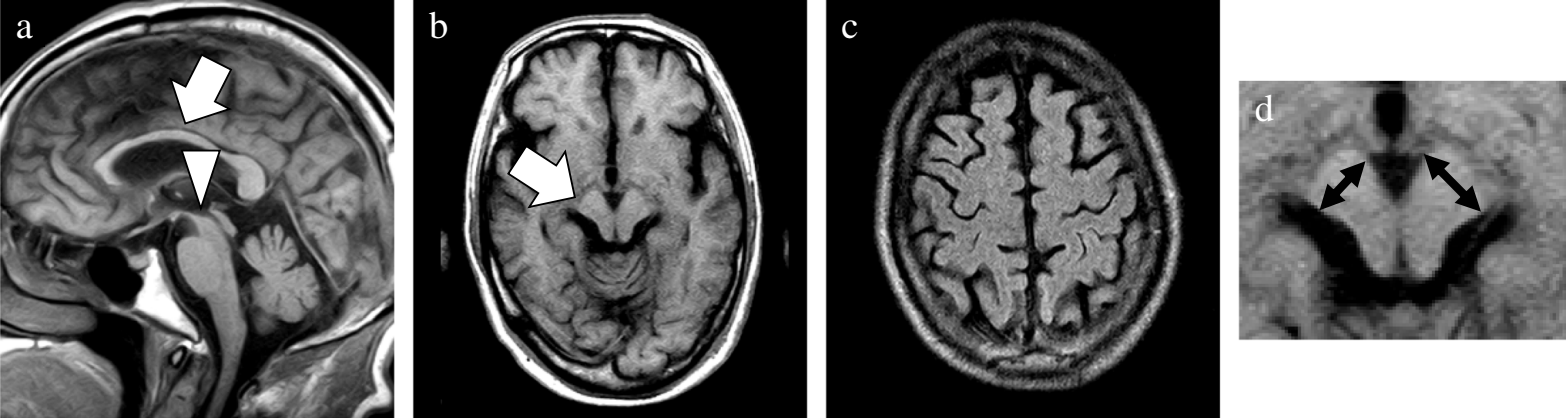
A representative case of CBD mimics. This 71-year-old male patient obtained a postmortem diagnosis of PSP. MRI was performed two years after the symptom onset. Sagittal and axial T1WI (a, b), and axial FLAIR image (c) showed atrophy of the midbrain and callosal body (arrow and arrowhead, a), and right dominant asymmetric atrophy of the cerebral peduncle without obvious SWMH (arrow, b). These MRI findings were very similar to those of the patient described in Fig. 6. The case of this patient was exhibited to clarify the challenges when differentiating CBD without SWMH from its mimics, especially PSP. A magnified view was exhibited to emphasize the asymmetry of the short axis in the cerebral peduncles (double sided arrows, d). a, sagittal T1WI; b, axial T1WI; c, axial FLAIR image; CBD, corticobasal degeneration; FLAIR, fluid attenuated inversion recovery; PSP, progressive supranuclear palsy; SWMH, subcortical white matter hyperintensity; T1WI, T1-weighted image
